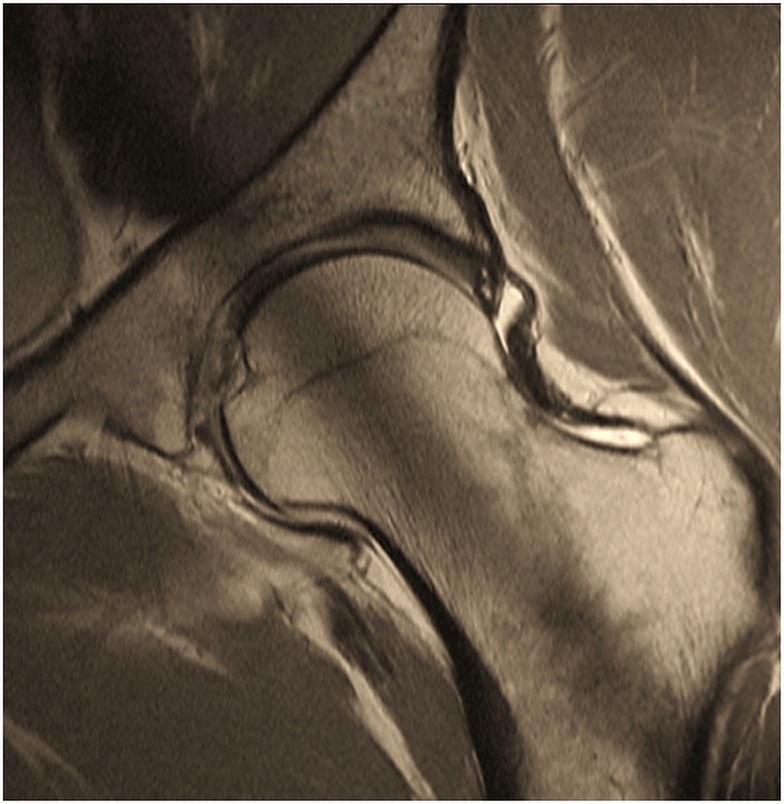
Two-dimensional proton-density (PD) – weighted MR image of a pincer-type FAI patient depicting an increased signal within the center of the labrum that does not extend to the labral margin reflecting intra-labral degeneration. Note that the saturation effect (band of low signal in the center of acetabulum and femoral neck) is constantly present in 2D radial MR imaging.
